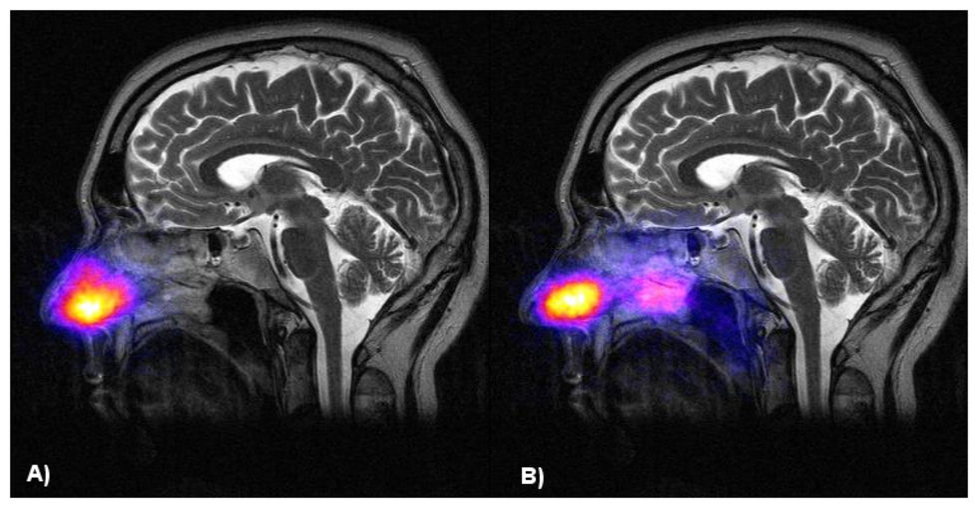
Comparison of deposition distribution of nasal pump spray and pulsating aerosol application. Lateral deposition distribution of nasal pump spray (A) and pulsating aerosol application (B) in a healthy volunteer (superposition of the lateral gamma camera image with an individual representative sagittal MRI slice).
